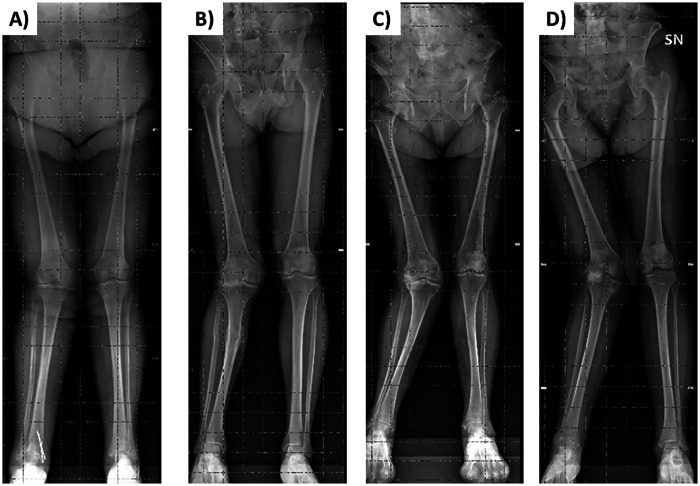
Weight-bearing anteroposterior radiographs of the entire lower extremities of 2 patients presenting grade II valgus deformity [tibiofemoral alignment for the right side of 11 ° in panel (A) and 13 ° in panel (B)] and of 2 patients presenting grade III valgus deformity [alignment for the right side of 22 ° in panel (C) and 25 ° in panel (D)].
